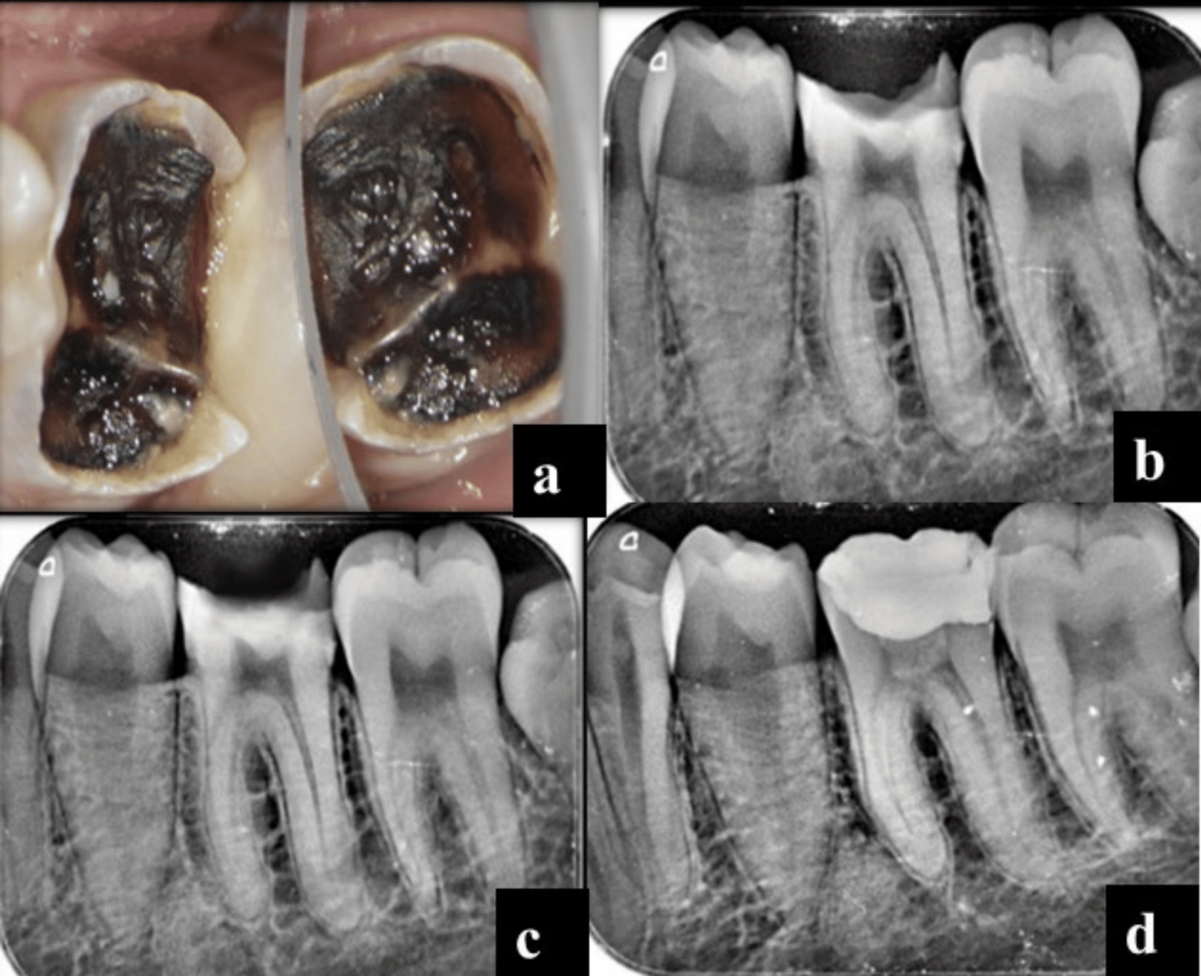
Preoperative clinical picture (a); preoperative radiograph (b); postoperative radiograph (c); 12-month follow-up (d)
